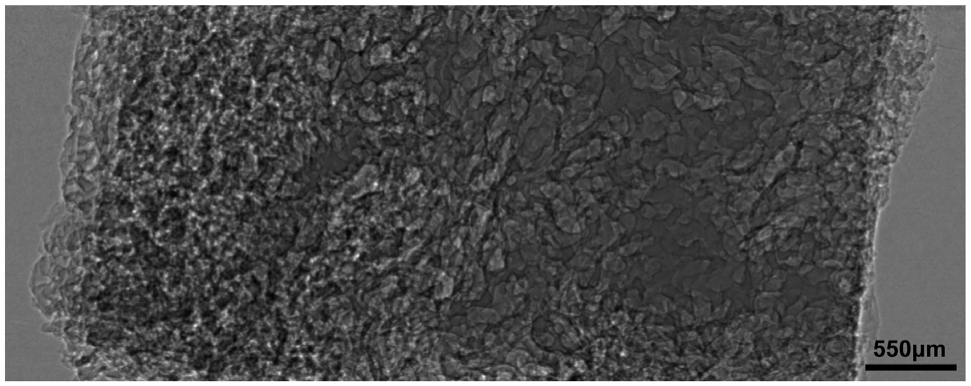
A planar x-ray image of the CHL sample. The hepatic sinusoids are clearly detected, but all of them are overlapped with each other.
